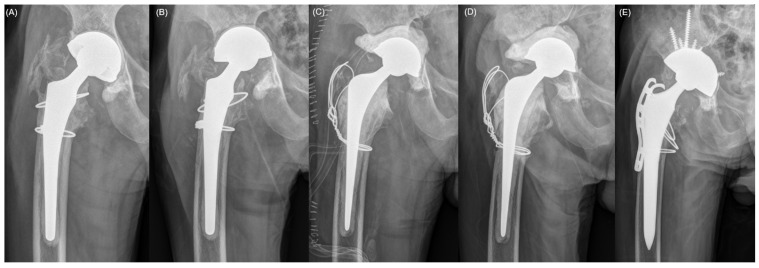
Recurrent cement mantle failure and subsequent revision in a 78-year-old male. (A) Immediate postoperative radiograph following the initial dual-mobility bearing (DMB)-incorporated functional articulating hip spacer (FAHS) implantation for periprosthetic joint infection. (B) Radiograph demonstrating the first mechanical failure characterized by medial displacement of the cement mantle into the acetabular defect. (C) Radiograph after a redo-FAHS procedure performed to restore joint continuity. (D) Radiograph showing a second cement mantle failure after the redo surgery. (E) Final radiograph following definitive revision total hip arthroplasty after infection eradication was achieved.
